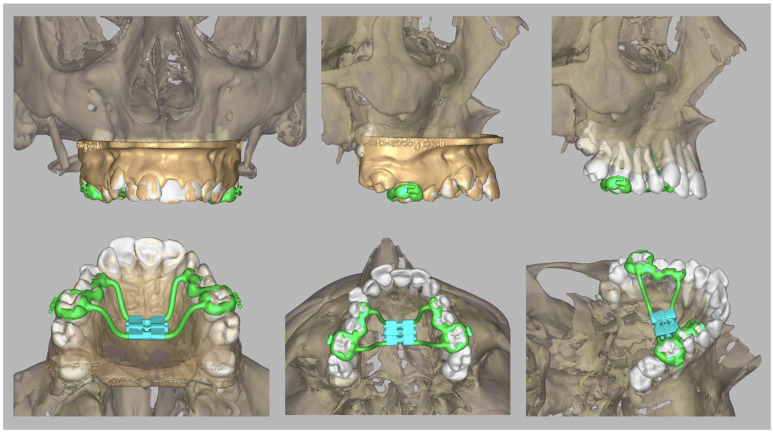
Digital design and plan of an expander and superimposition of the intraoral scan and CBCT image using Exocad DentalCAD 3.0 Galway software.
